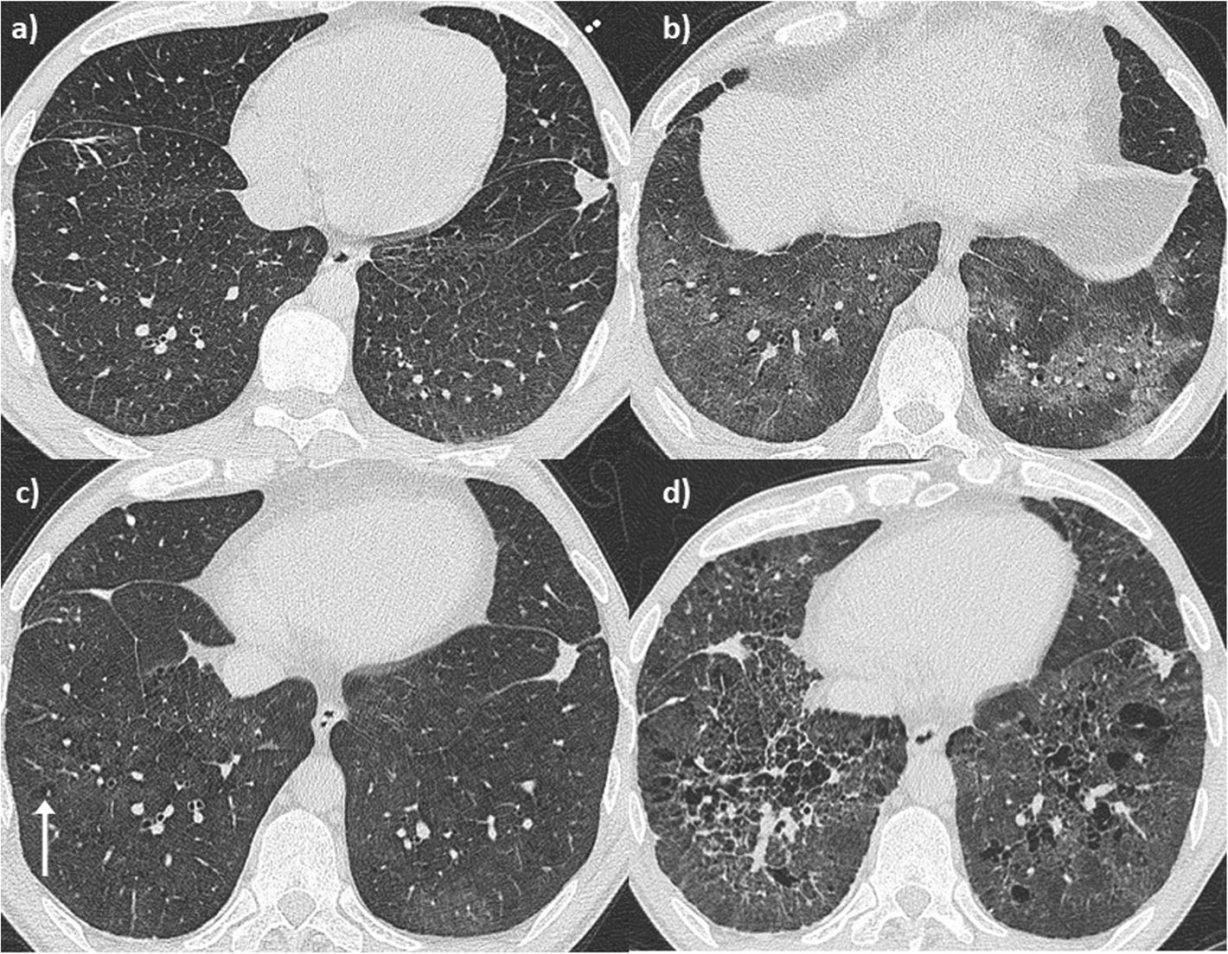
Patient 2. Radiological evolution. Axial CT images of the lung. a CT scan at first presentation (34 years old) demonstrates a subtle ground glass infiltrate, smooth interlobular septal thickening and a focal nodule in the subpleural left lower lobe. b At 40 years old, imaging reveals a more obvious ground glass infiltrate. c At 42 years old, perivascular emphysema/cysts are evident (white arrow), which d subsequently progresses to emphysema 2 years later. Similarly to Fig. 1 d this has a perivascular distribution
